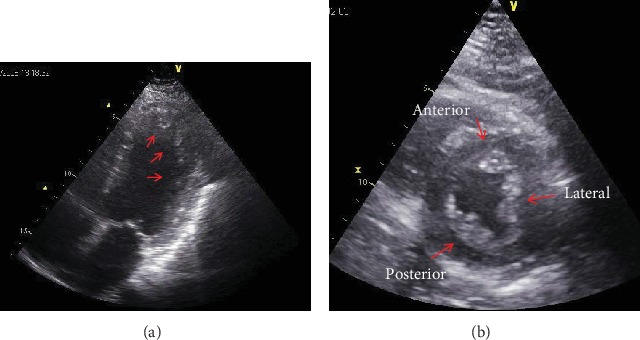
Echocardiographic apical 4-chamber view showing left ventricular hypertrabeculation/noncompaction affecting the apex of the left ventricle (a). Echocardiographic short axis view of the left ventricle with hypertrabeculation/noncompaction affecting the anterior, lateral, and posterior walls (b).
